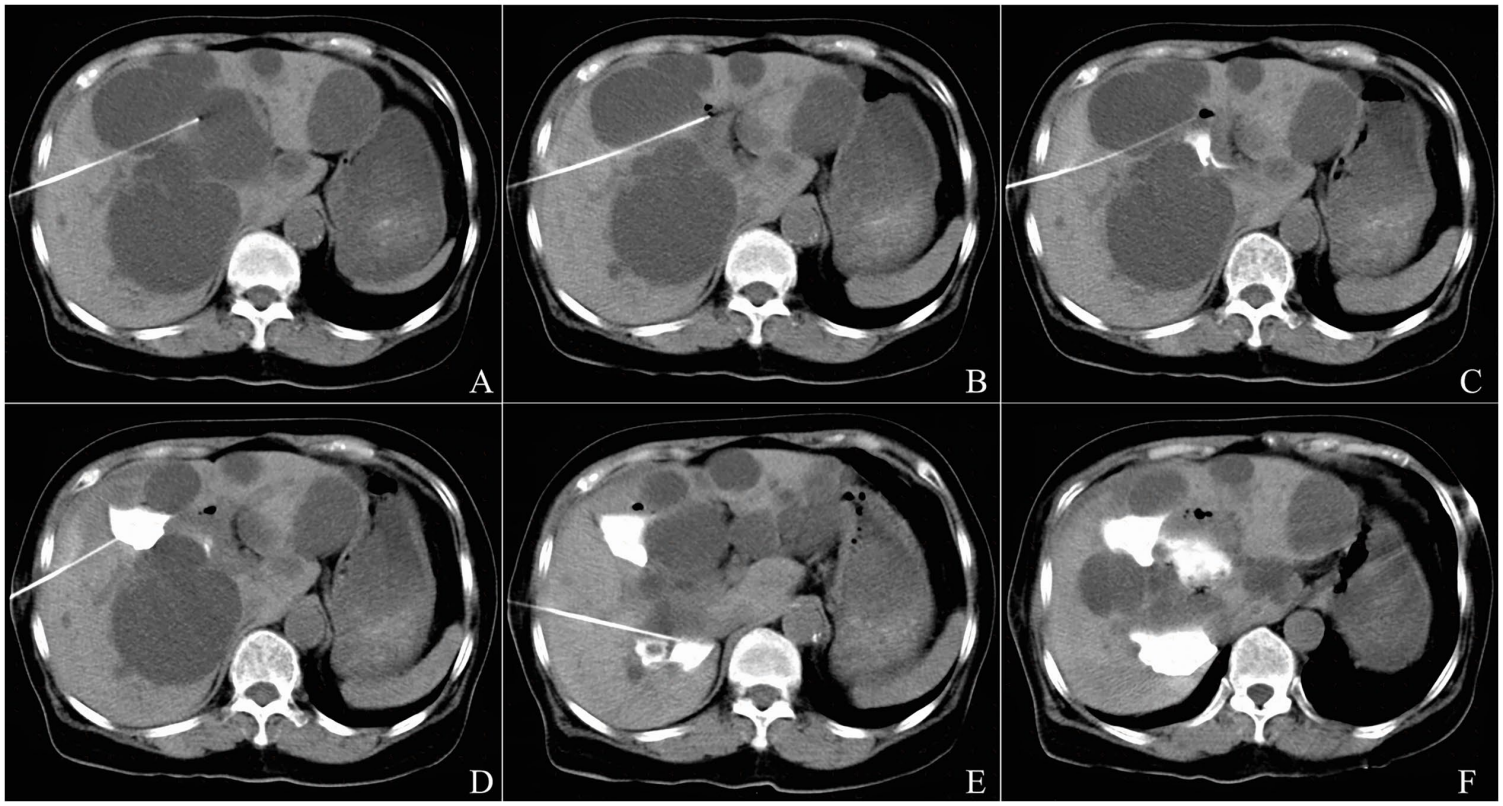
The single-point multi-angulated puncturing technique of CT-guided percutaneous needle aspiration and bleomycin sclerotherapy for three cysts in a 70-year-old female patient with polycystic liver disease. A 15-cm 21-gage Chiba needle was inserted toward the ventral orientation from the right midaxillary line, and went through the cyst located in the lateral section of liver segment V and entered into the cyst located in the medial section of liver segment V (A). The cyst located in the medial section of liver segment V was evacuated completely (B), and bleomycin solution contained diluted contrast media was injected into the cyst cavity without evidence of leakage (C). The needle was then withdrawn into the cyst located in the lateral section of liver segment V, the procedure of aspiration and sclerotherapy was repeated (D). Next, the needle was drawn back to the subcutaneous layer, and than was inserted toward the dorsal orientation from the right midaxillary line and entered into the cyst located in liver segment VI; the procedure of aspiration and sclerotherapy was performed (E). The postprocedure CT scan revealed that bleomycin solution remained behind in three cysts without evidence of spillage (F).
